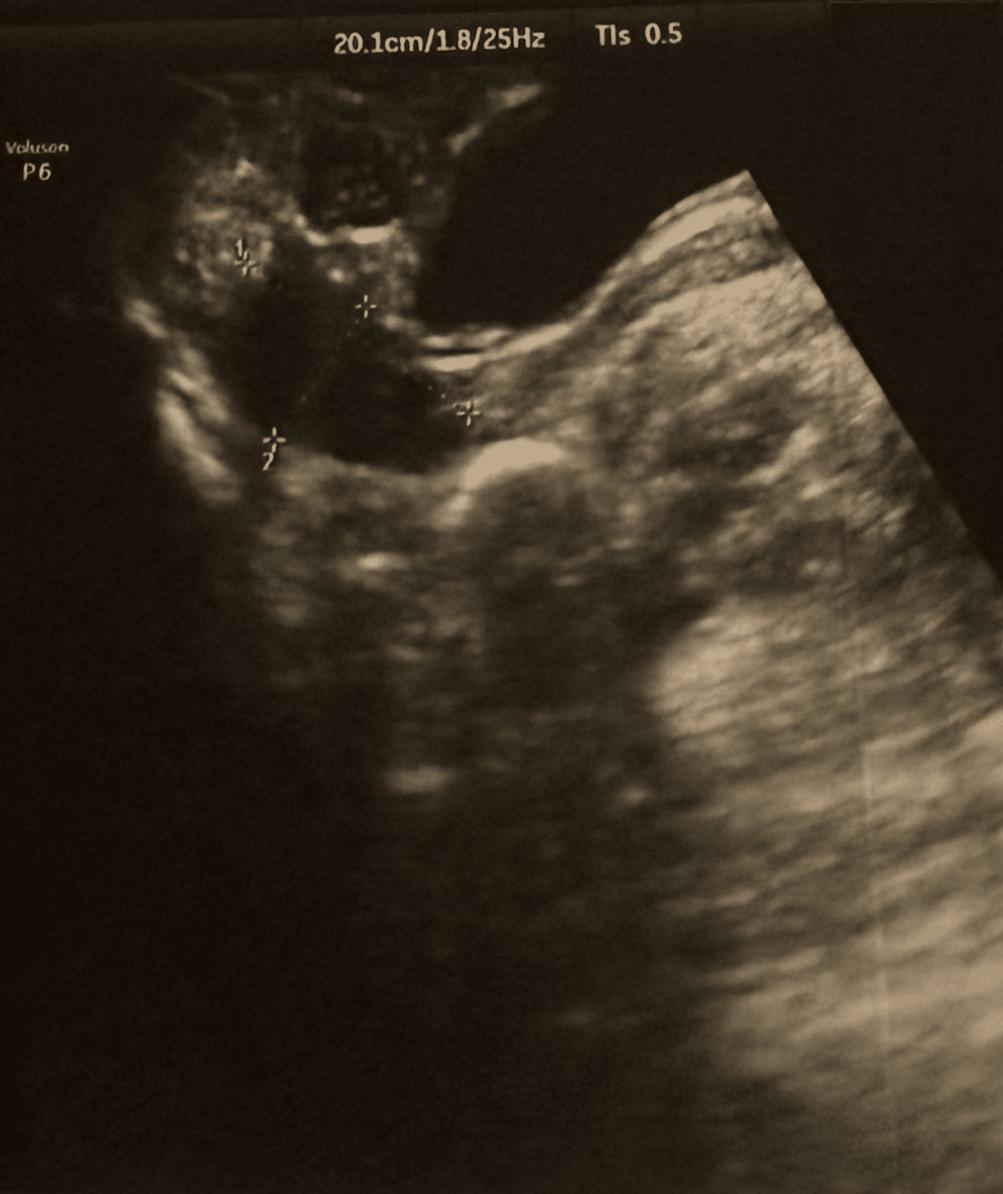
Axillary ultrasound image showing left axillary lymphadenopathy This image shows the left axillary lymphadenopathy measuring 3.8 cm in its long axis, with an oval shape.
